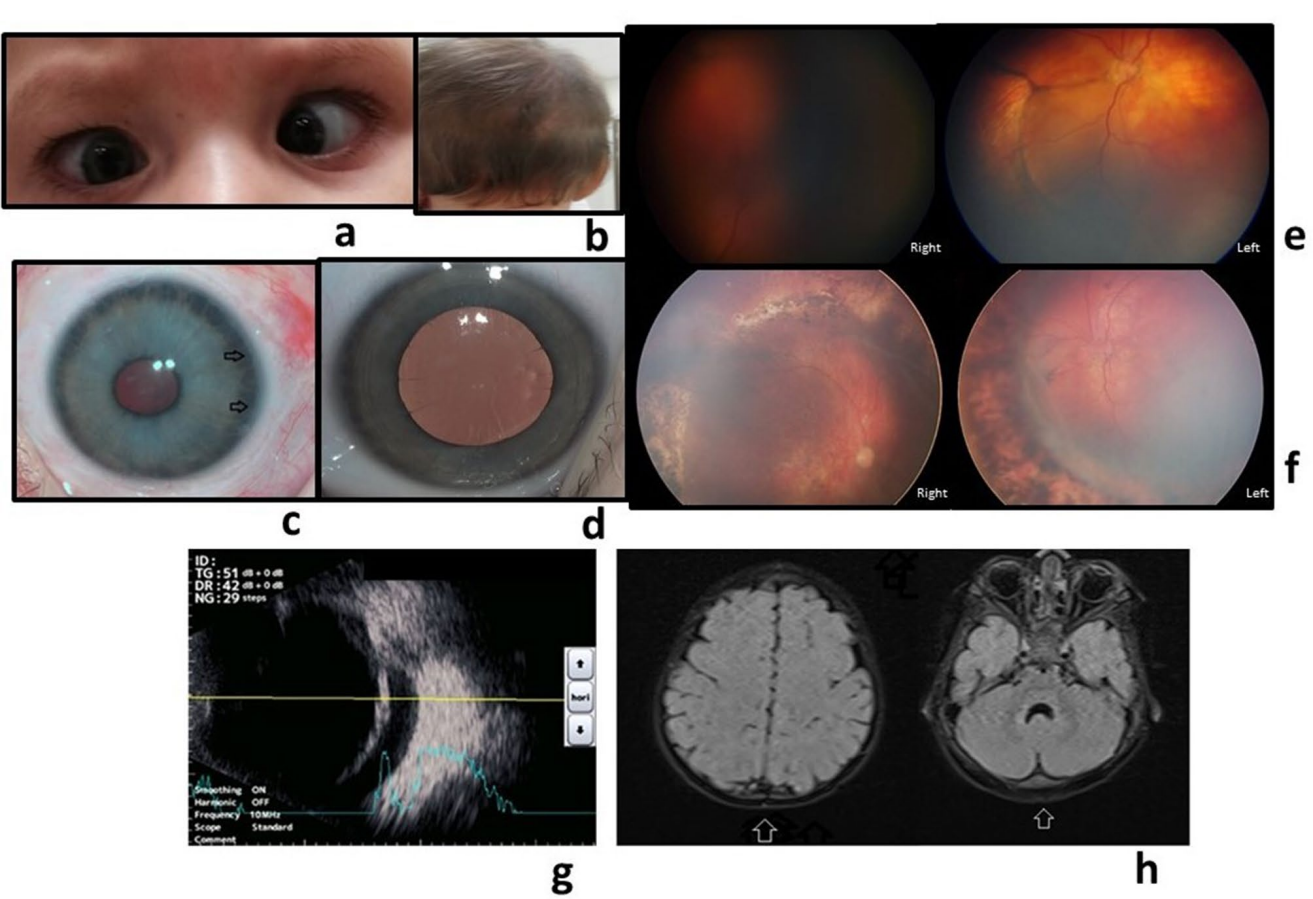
a-alternate esotropia b-occipital fat pad c-Right eye pupil, iris, and lens (arrows indicate posterior embryotoxon) d- Left eye pupil, iris and lens e- Fundus photo of right and left eye, chorioretinal hyperpigmented demarcation line on the peripheral retina, and shallow serous retinal detachment (The right fundus footage was not adequately illuminated due to poor pupil dilatation) f-Fundus views after scleral buckling, pars plana vitrectomy (PPV), and endolaser g- Serous detachment on B-sacan ultrasonography (USG) h- Cranial axial T1-weighted (T1W) magnetic resonance imaging: Occipital fat pad and midline occipital defect
